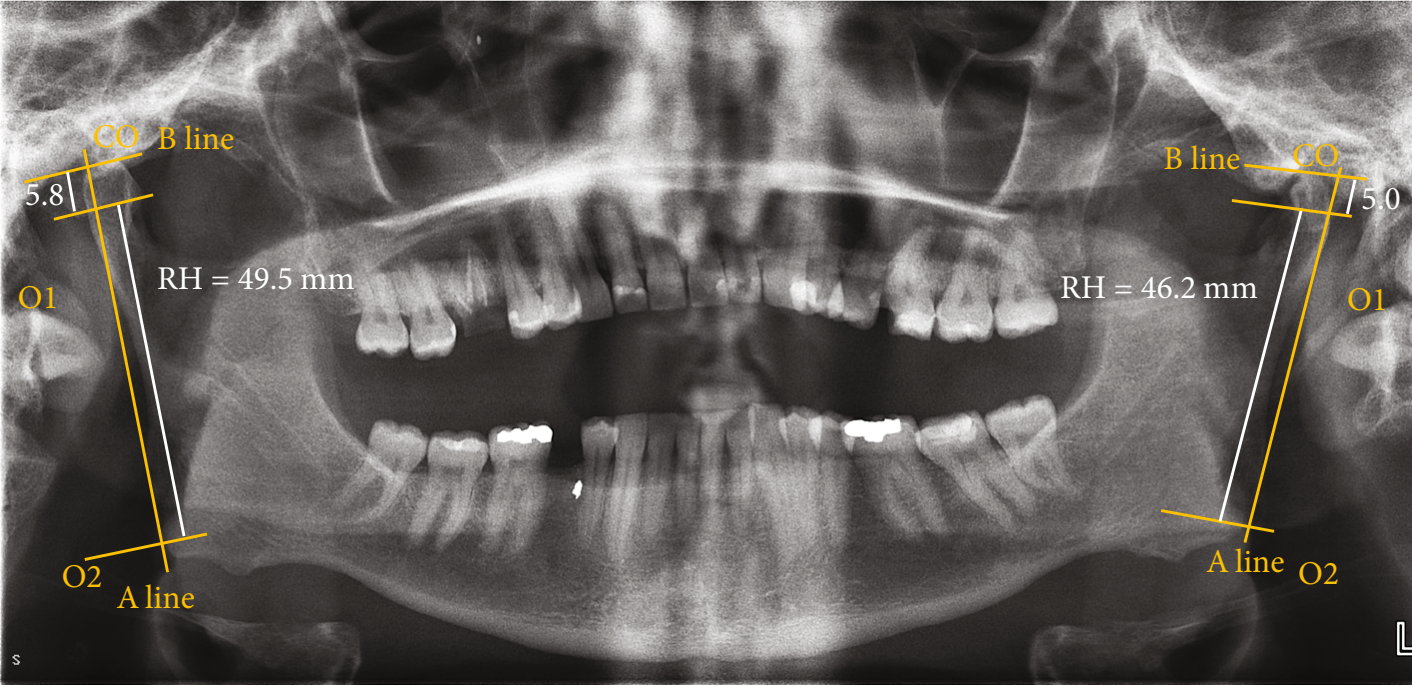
Orthopantomography of the patient. Left coronoid process hyperplastic (yellow arrow). Left condyle hypoplastic (red arrow).
